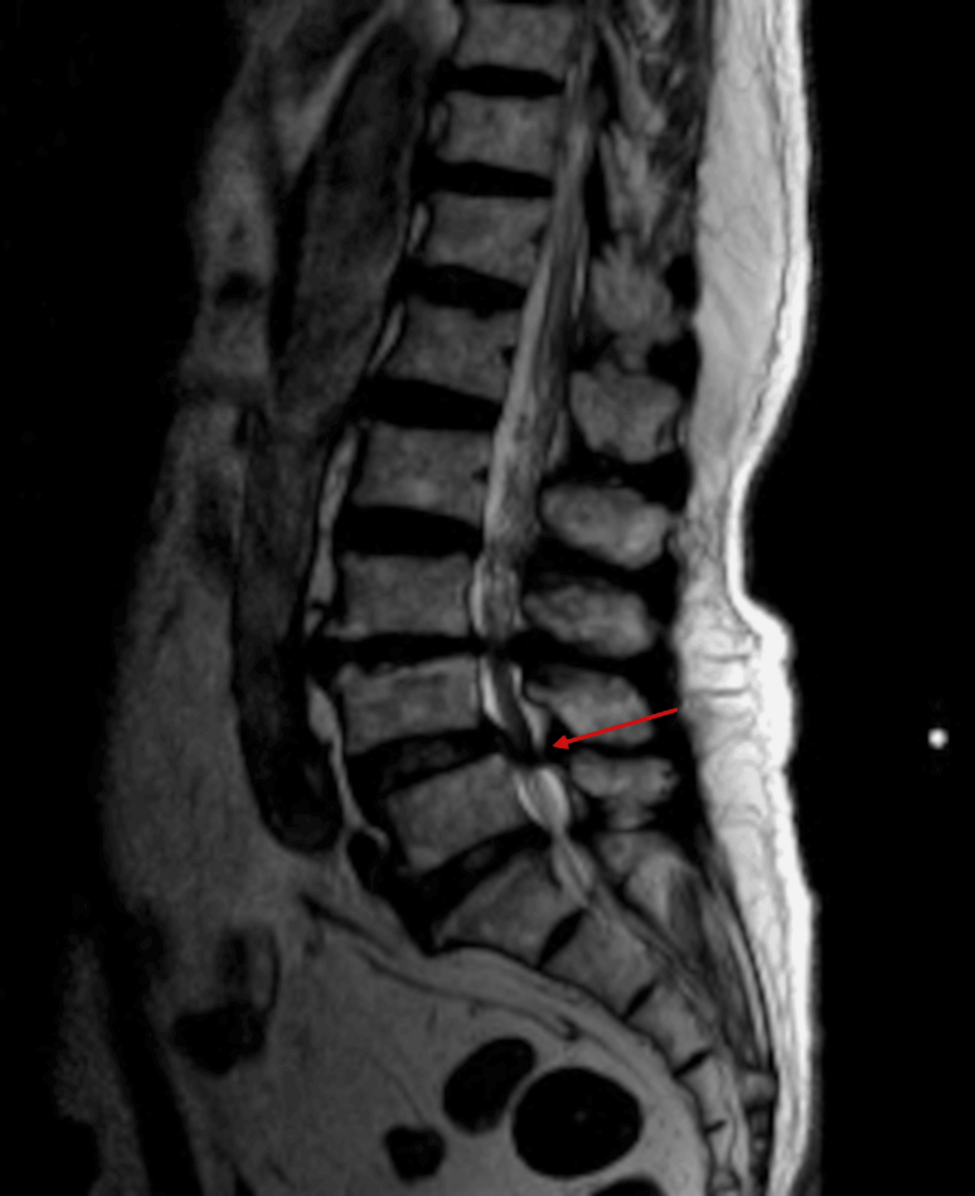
Lumbar spine MRI (T2) taken before the procedure (sagittal view). The red arrow indicates the area of severe central stenosis at the L4/5 level. Sagittal view of lumbar spine magnetic resonance imaging (L-MRI) showing severe central stenosis at the L4/5 level, as indicated by the red arrow.
